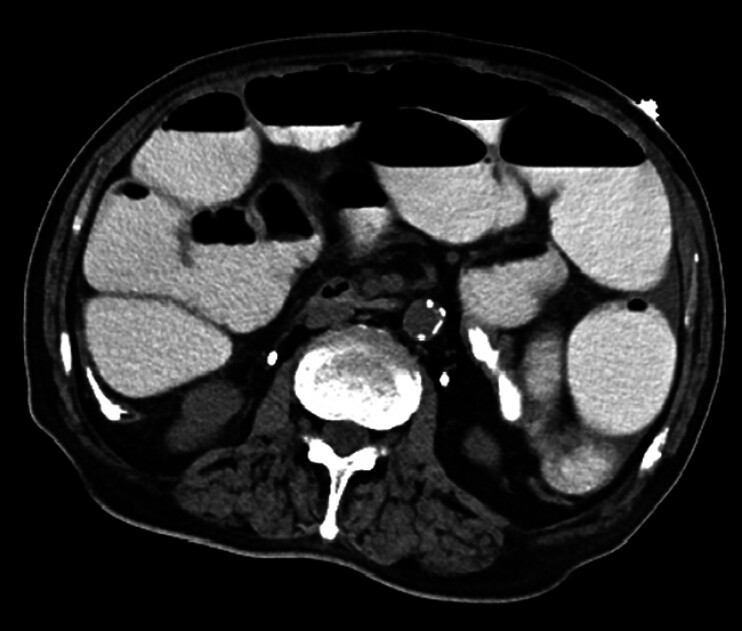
The abdominal CT imaging revealed incomplete small bowel obstruction. CT, computed tomography.
